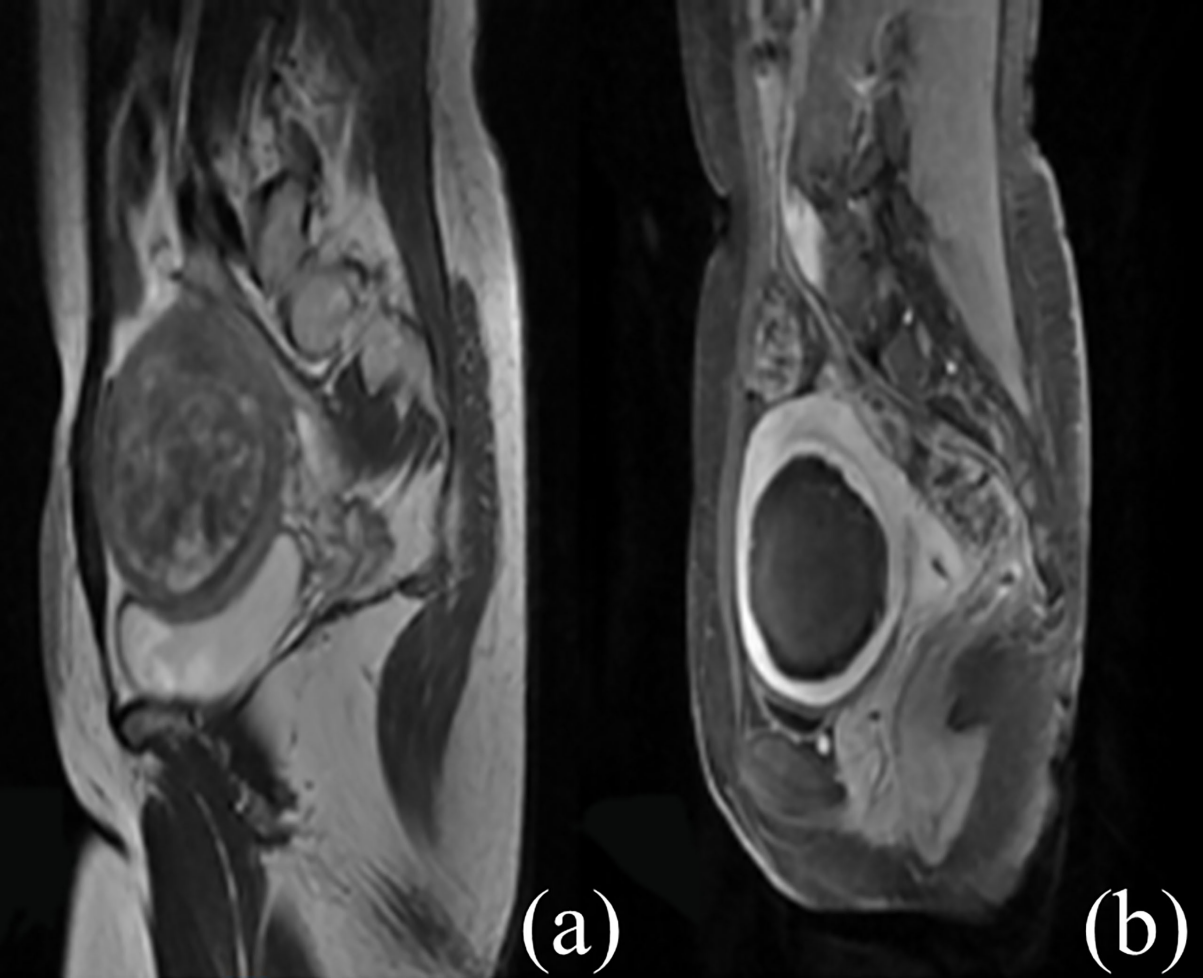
Contrast-enhanced MR images before and after HIFU treatment. (A) The fibroid was subserous, anterior, and hyperintense before treatment. (B) The non-perfused volume was shown inside uterine fibroid after treatment.
